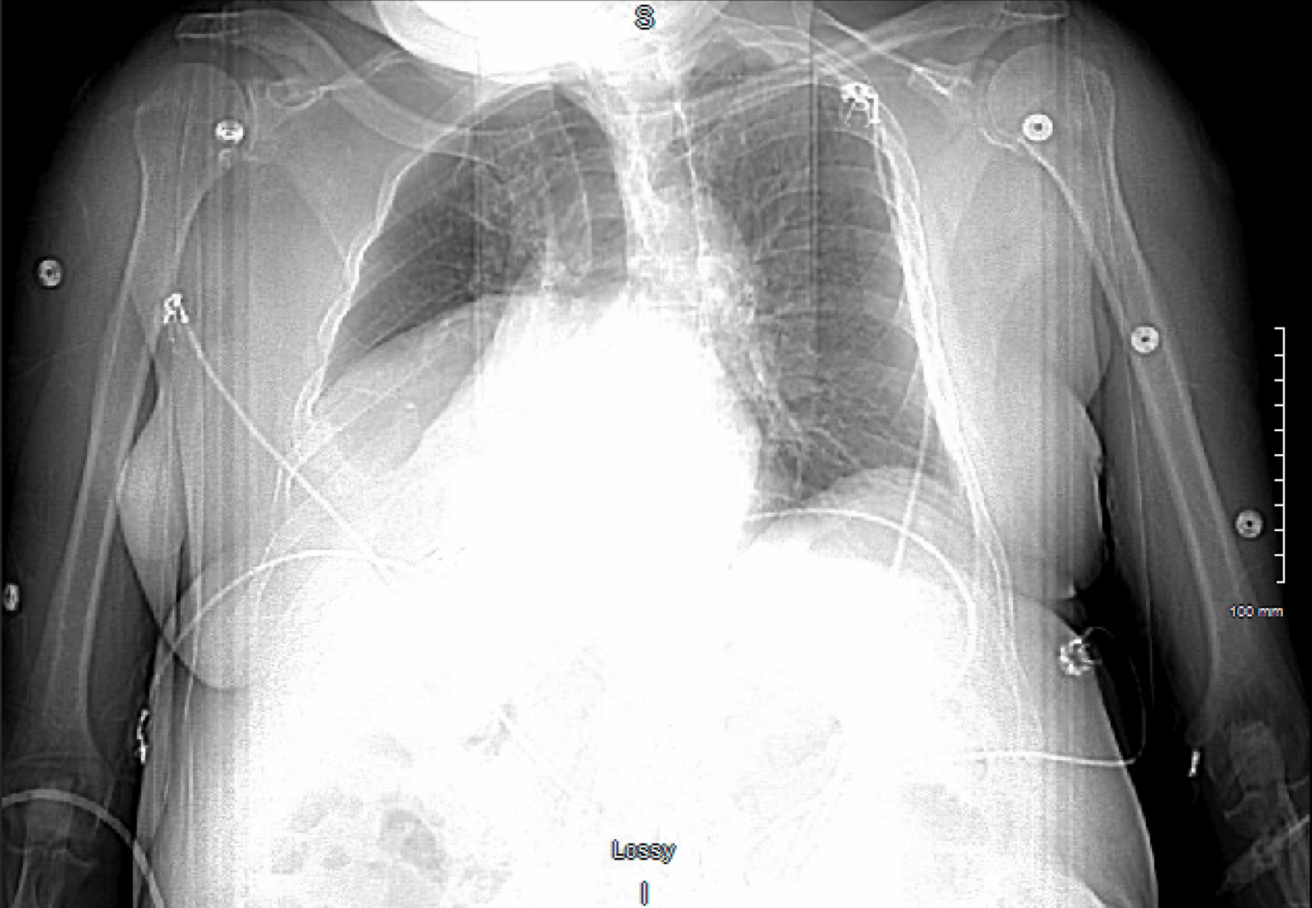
Chest X-ray demonstrating no evidence of acute cardiopulmonary disease
